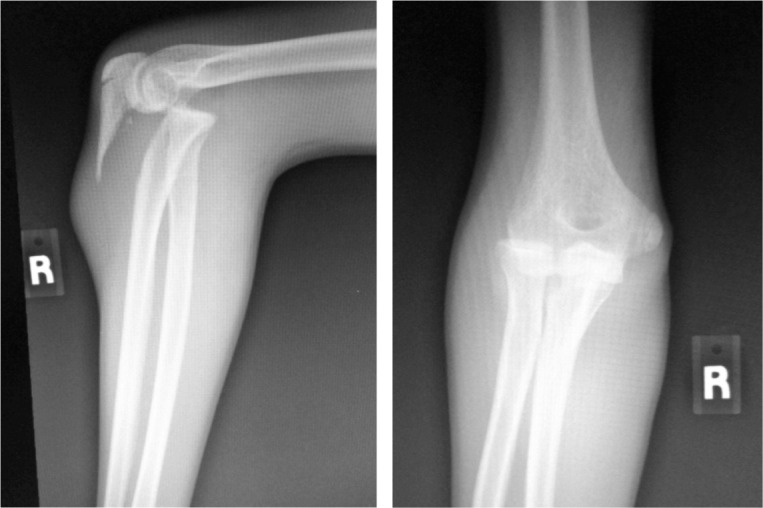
Initial radiograph taken in emergency department showing proximal ulna fracture with radial head anterior dislocation. Fracture of proximal ulna extending to olecranon articular surface.
